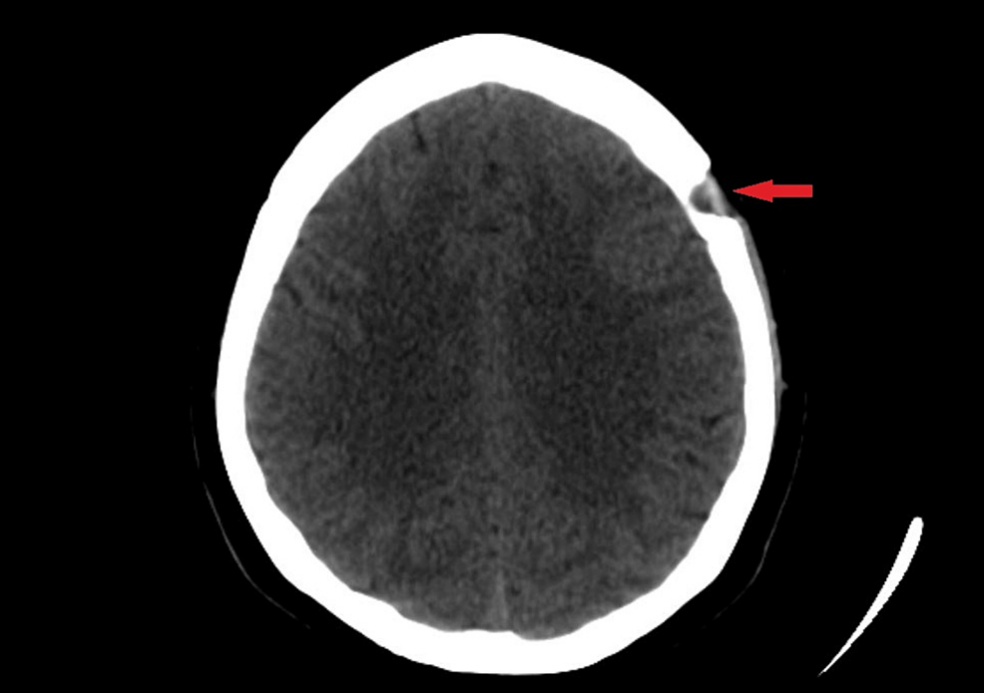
Postoperative CT brain showing complete evacuation of left SDH Arrow shows the location of the left frontal burr hole. SDH - subdural hematoma
